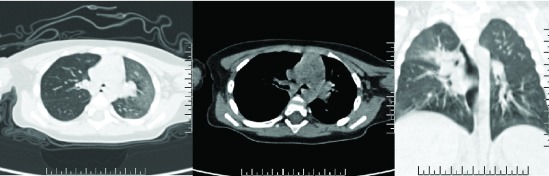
CT scan of the chest showing increased right lung volume and increased transparency of lung field. There is soft tissue density shadow seen in the right main bronchus blocking the lumen. The trachea is shifted slightly to the left, but no obvious abnormity is seen. The chest CT was suggestive of right bronchial foreign body, with right sided obstructive emphysema.
